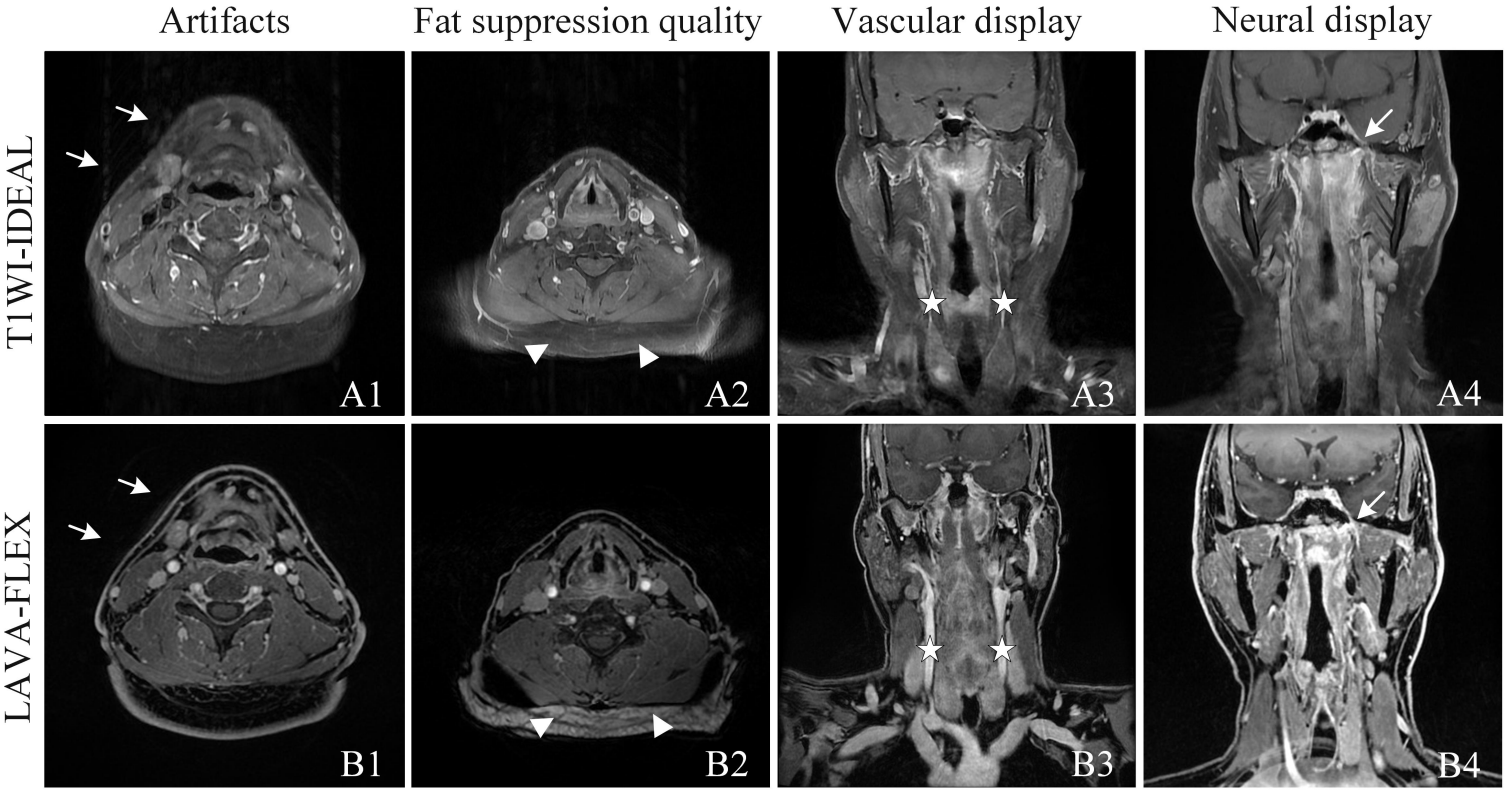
Qualitative analysis of T1WI-IDEAL (A) and LAVA-Flex (B) images of NPC. Both T1WI-IDEAL and LAVA-Flex MR images demonstrated excellent image quality, receiving a rating of five points. However, both readers noted that the LAVA-Flex sequence exhibited fewer artifacts (A1, B1, indicated by arrows) and provided superior visualization of vessels (A3, B3, indicated by pentagrams) and nerves (A4, B4, indicated by arrows) compared to T1WI-IDEAL. On the other hand, T1WI-IDEAL (A2) achieved stronger fat suppression (indicated by a triangle) than LAVA-Flex (B2).
